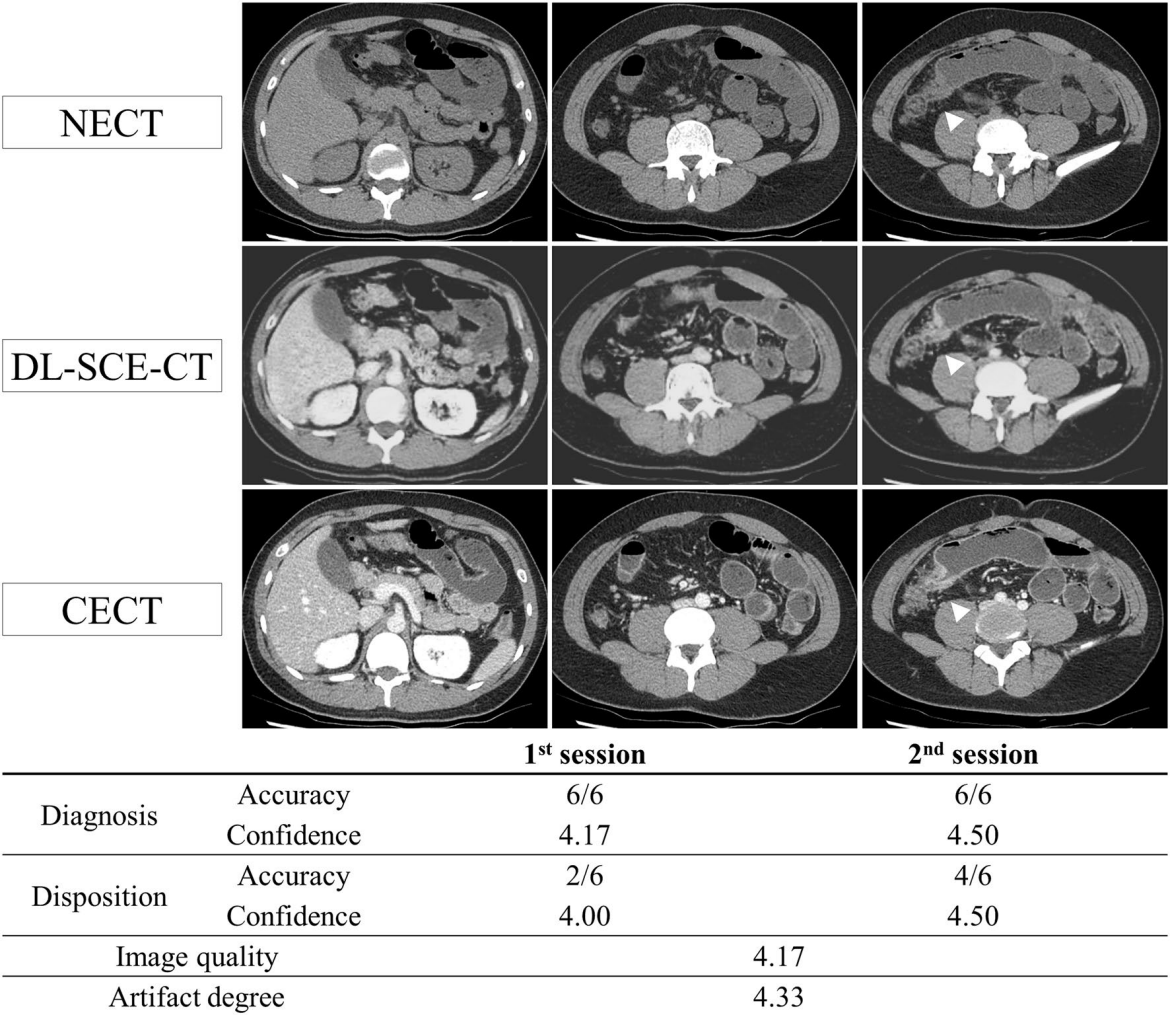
A 25-year-old male patient who visited the ED complaining of abdominal pain. CT images show fluid distension of small bowel loops with transition at the terminal ileum (arrowhead). The contrast among the bowel wall, visceral fat, and intraluminal fluid is more evident in DL-SCE-CT than in NECT. The patient was admitted for management of Crohn’s disease flares. In this case, all of reviewers made the correct diagnosis (small bowel obstruction at terminal ileum) regardless of DL-SCE-CT. However, two more radiologists made correct disposition decision (admission for medical management) after review of DL-SCE-CT. Moreover, with the aid of DL-SCE-CT, the confidence of the diagnosis and disposition decision increased from 4.17 to 4.50 and 4.00 to 4.50, respectively.
